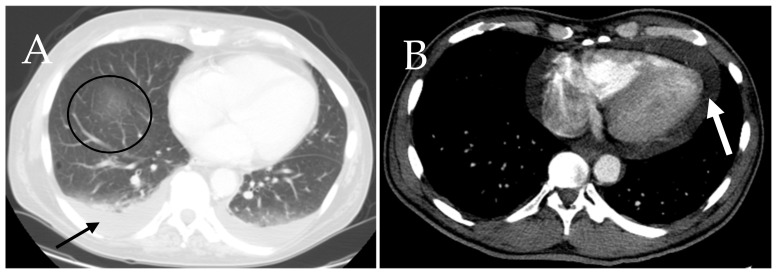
A 64-year-old male with mastocytosis. Axial contrast-enhanced CT scan of thorax demonstrates (A) areas of fluid at the base of the lung denoting pleural effusion (black arrow), (B) fluid surrounding the heart as an evidence of pericardial effusion (white arrow) and focal pulmonary ground glass opacity (circle).
